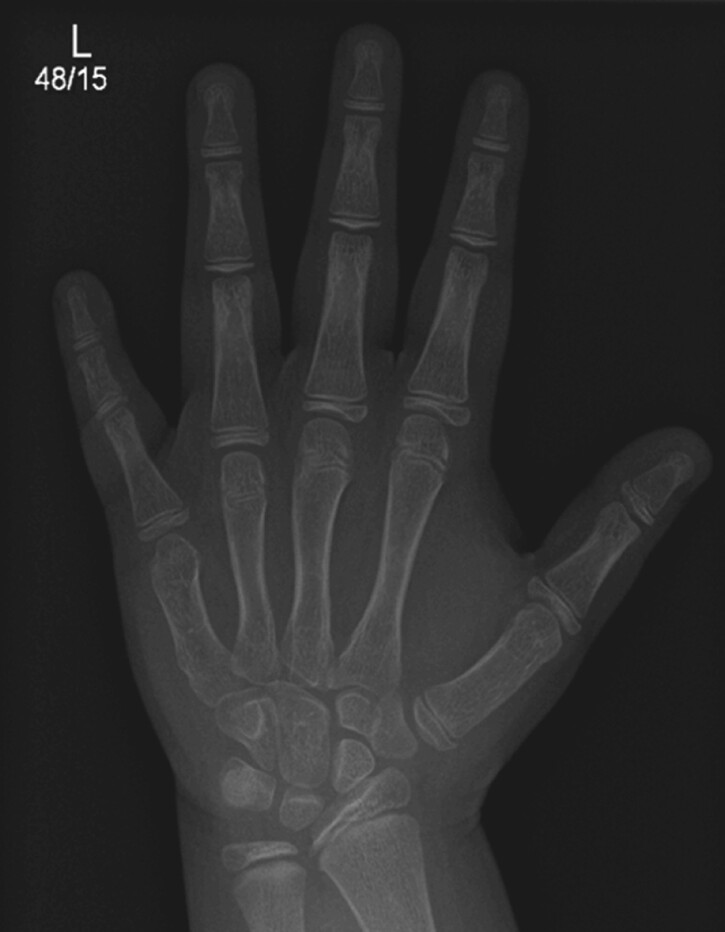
X-ray of the hand and wrist of case 1 showing mild brachydactyly with short 5th metacarpal.
